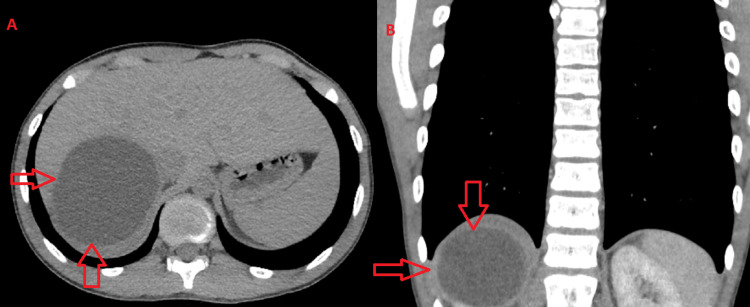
Preoperative abdominal CT scan A: CT scan; axial plane. B: CT scan; coronal plane
